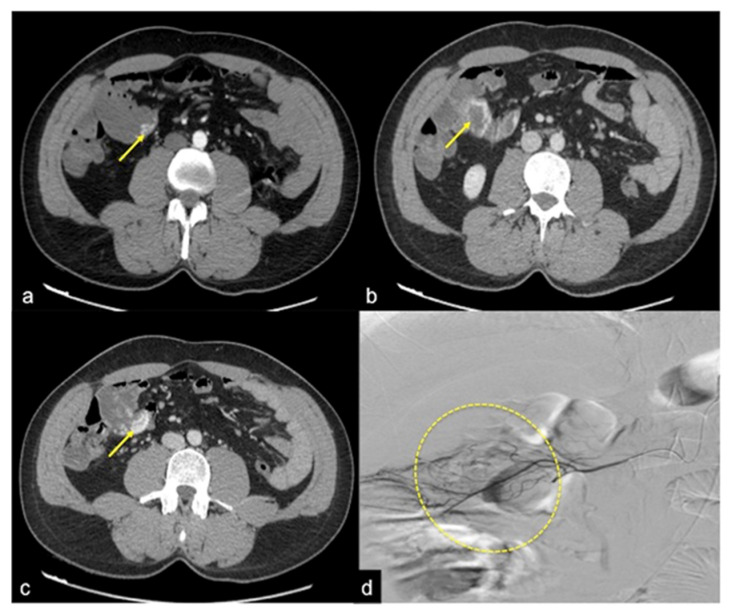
CTA multiphasic study of small intestine angiodysplasia. In the arterial phase (a) an active blush of contrast media is detected at the small bowel wall (arrow). The venous (b) and late (c) phases show a gradual increase of contrast blush (arrow (b)) with consequent production of a haematic collection (arrow (c)). In the selective angiography (d) the CTA findings are confirmed and the presence of an angiodyslastic focus is documented (discontinued circle).
